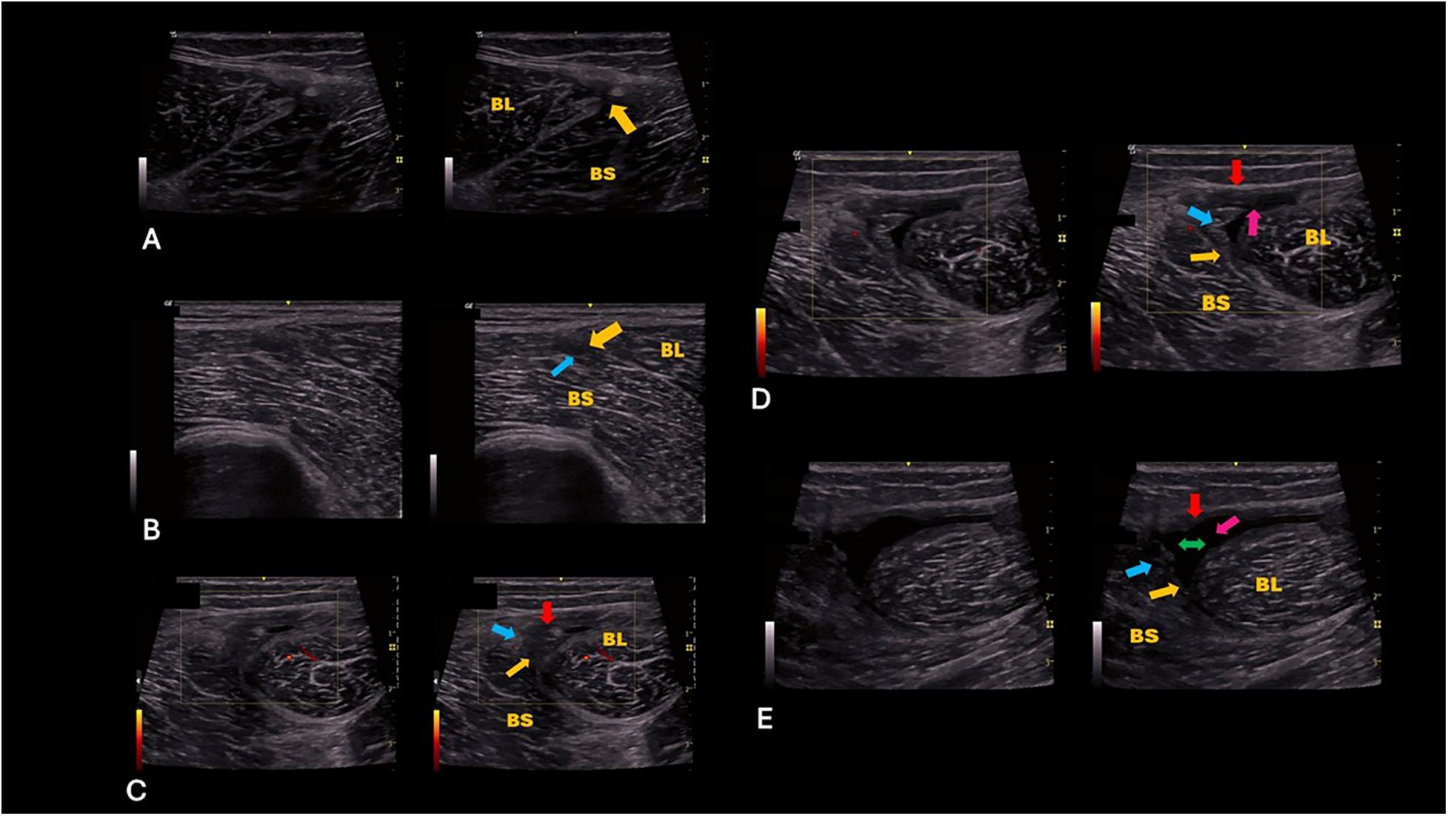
(A) A discontinuity to the deep myo-aponeurotic connective tissue component of the T-junction (yellow arrow). (B) A discontinuity to the deep myo-aponeurotic connective tissue component of the T-junction (yellow arrow), Extension of the discontinuity into the myofascial connective tissue of the Biceps Femoris short head (blue arrow). (C) A discontinuity to the deep myo-aponeurotic connective tissue component of the T-junction (yellow arrow), A discontinuity to the superficial myo-tendinous connective tissue component of the T-junction (red arrow), Extension of the discontinuity into the myofascial connective tissue of the Biceps Femoris short head (blue arrow). (D) A discontinuity to the deep myo-aponeurotic connective tissue component of the T-junction (yellow arrow), A discontinuity to the superficial myo-tendinous connective tissue component of the T-junction (red arrow), Extension of the discontinuity into the myofascial connective tissue of the Biceps Femoris short head (blue arrow), Formation of an intermuscular haematoma between the long and short heads of the biceps femoris (pink arrow). (E) A discontinuity to the deep myo-aponeurotic connective tissue component of the T-junction (yellow arrow), A discontinuity to the superficial myo-tendinous connective tissue component of the T-junction (red arrow), Extension of the discontinuity into the myofascial connective tissue of the Biceps Femoris short head (blue arrow), Formation of an intermuscular haematoma between the long and short heads of the biceps femoris (pink arrow), Asynchronous movement (gapping/separation) of the long and short heads of the biceps femoris on dynamic ultrasound with a resisted isometric knee flexor contraction. BL, Biceps Femoris long head; BS, Biceps Femoris short head. (Created by the authors).
